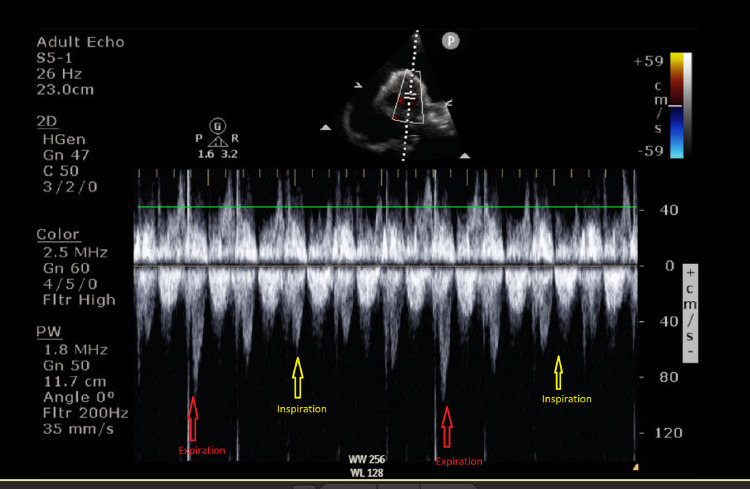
Transthoracic echo Variability with respiration at the left ventricular outflow tract (pulsed-wave Doppler).
